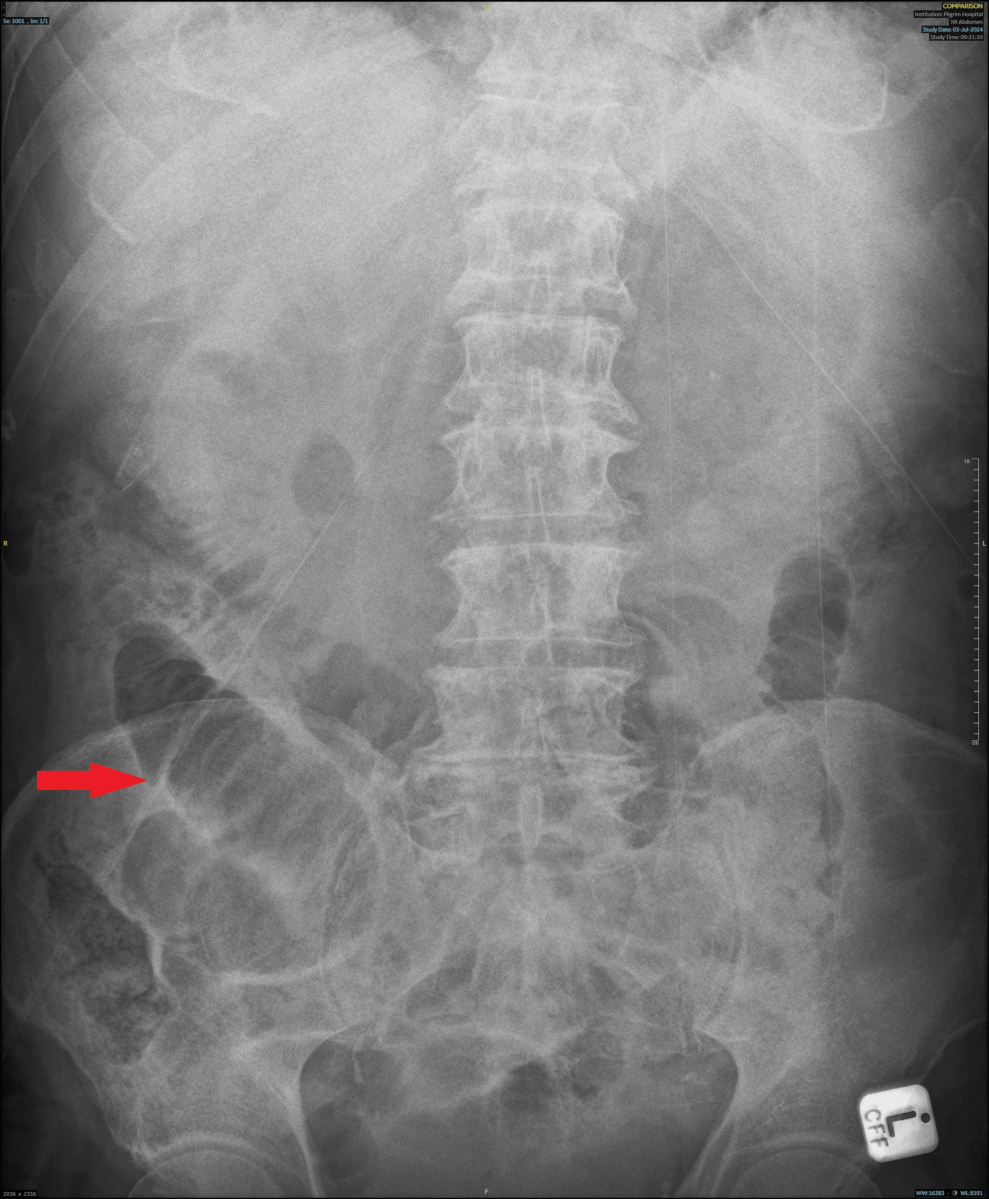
Abdominal X-ray. Dilated gas filled loops of small bowel measuring up to 4.2 cm are seen in the right flank.
